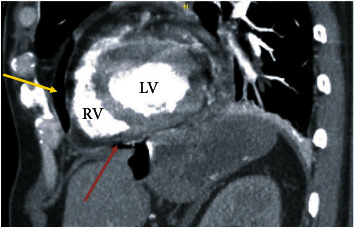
Sagittal view of chest CT showing pneumopericardium (yellow arrow) and gastropericardial fistula (red arrow).
